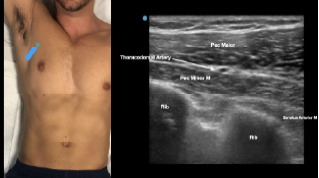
Final probe placement prior to the pectoralis nerve block I and II: blue line indicates transducer, and green dot indicates directional marker corresponding to ultrasound image.
